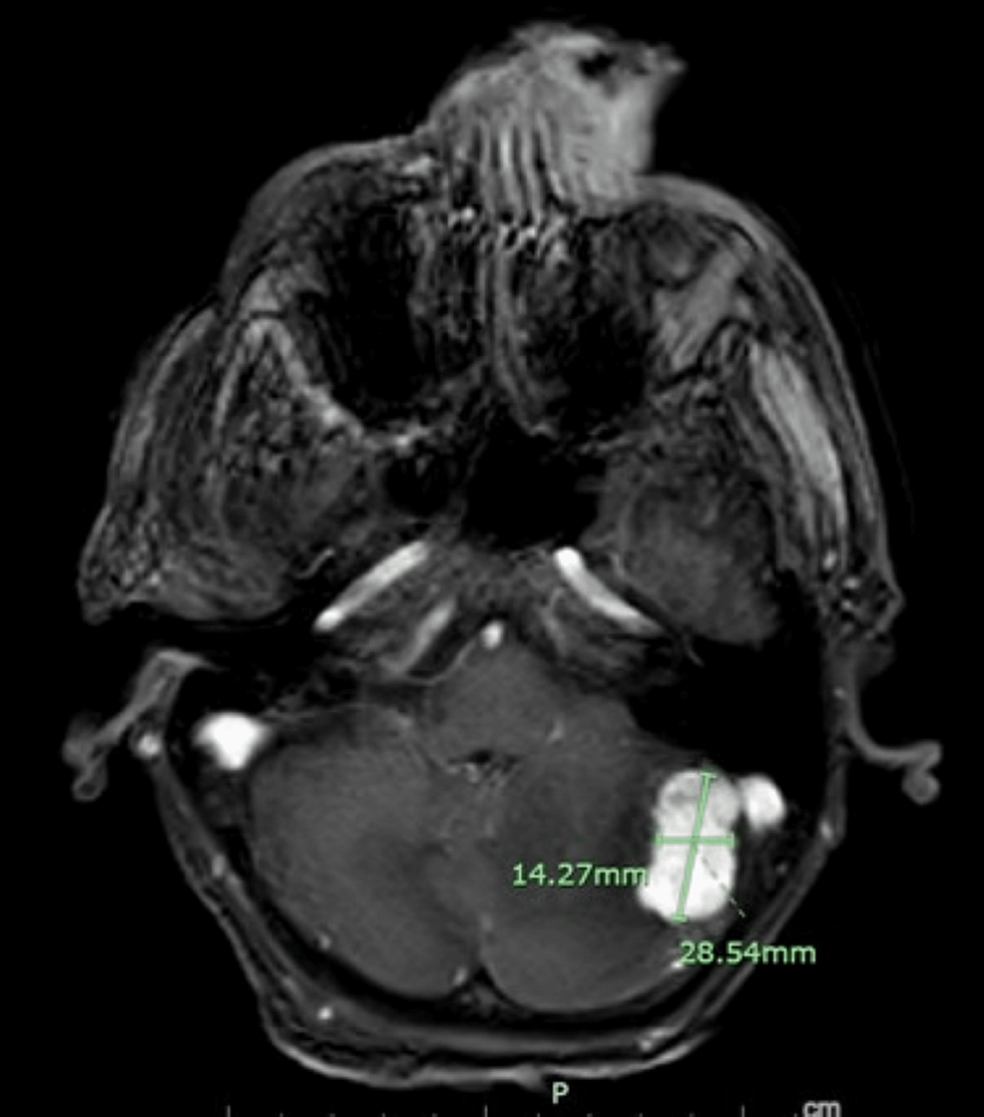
Metastatic cerebellar mass The MRI T1-weighted FFE image shows the enhancing 2.8 cm left cerebellar hemisphere mass. FFE: Fast field echo
